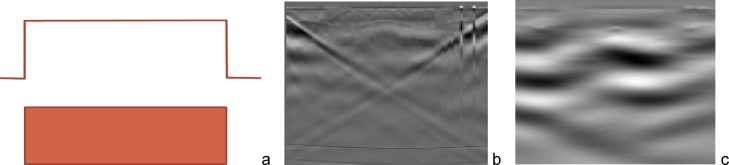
(a) Sharp rectangular profile of the optical beam from an optoacoustic hand-held probe causes V-shaped artefact on the sonogram of optoacoustic signals, and (b) low frequency wavy artefact objects on corresponding optoacoustic images.
